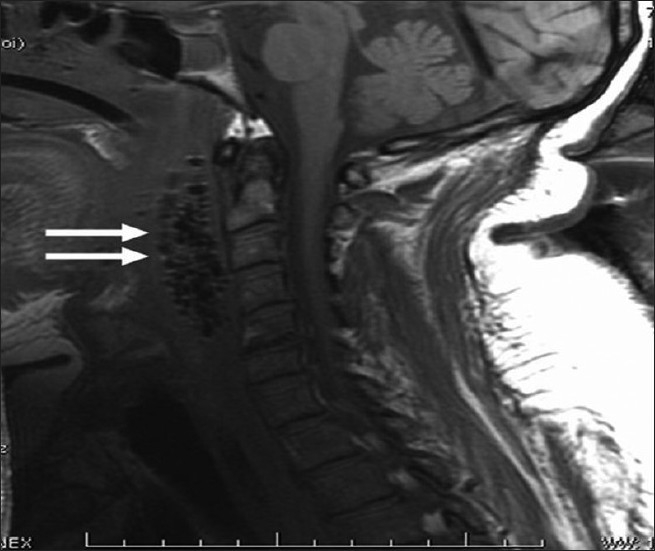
A sagittal T1 cervical MRI reveals air and air-bubble accumulation in the dangerous space (two arrows)
